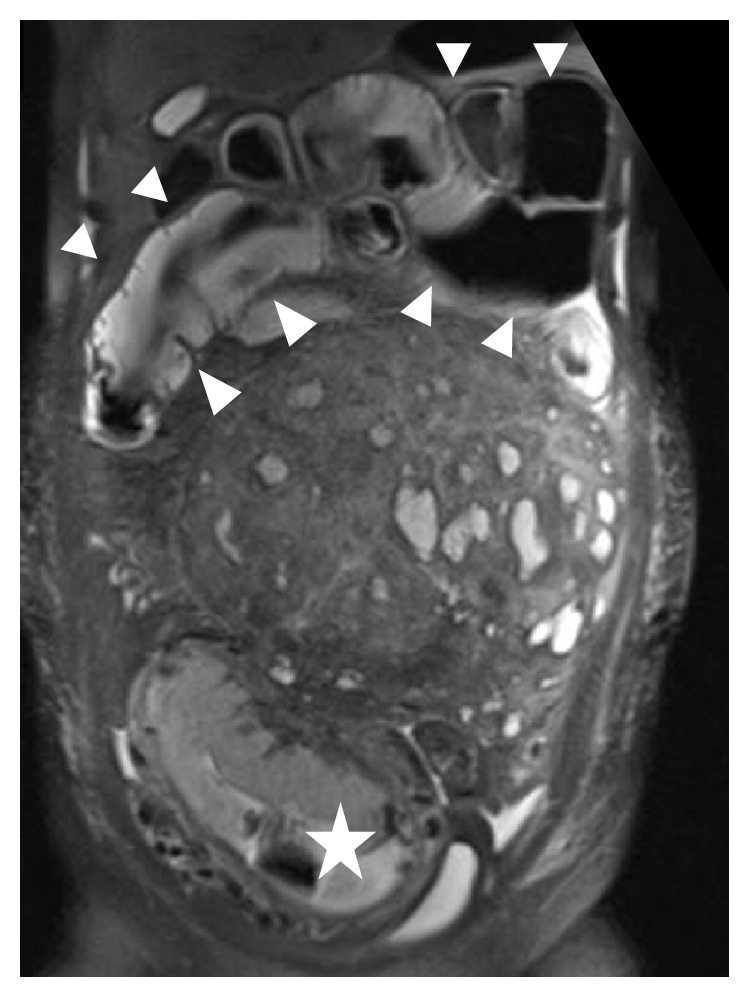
Magnetic resonance imaging of this patient at 12^6/7^ weeks of gestation. Coronal T2-weighted image shows the uterus (star). A large myoma and a dilated intestinal tract (arrowhead) are visible.
